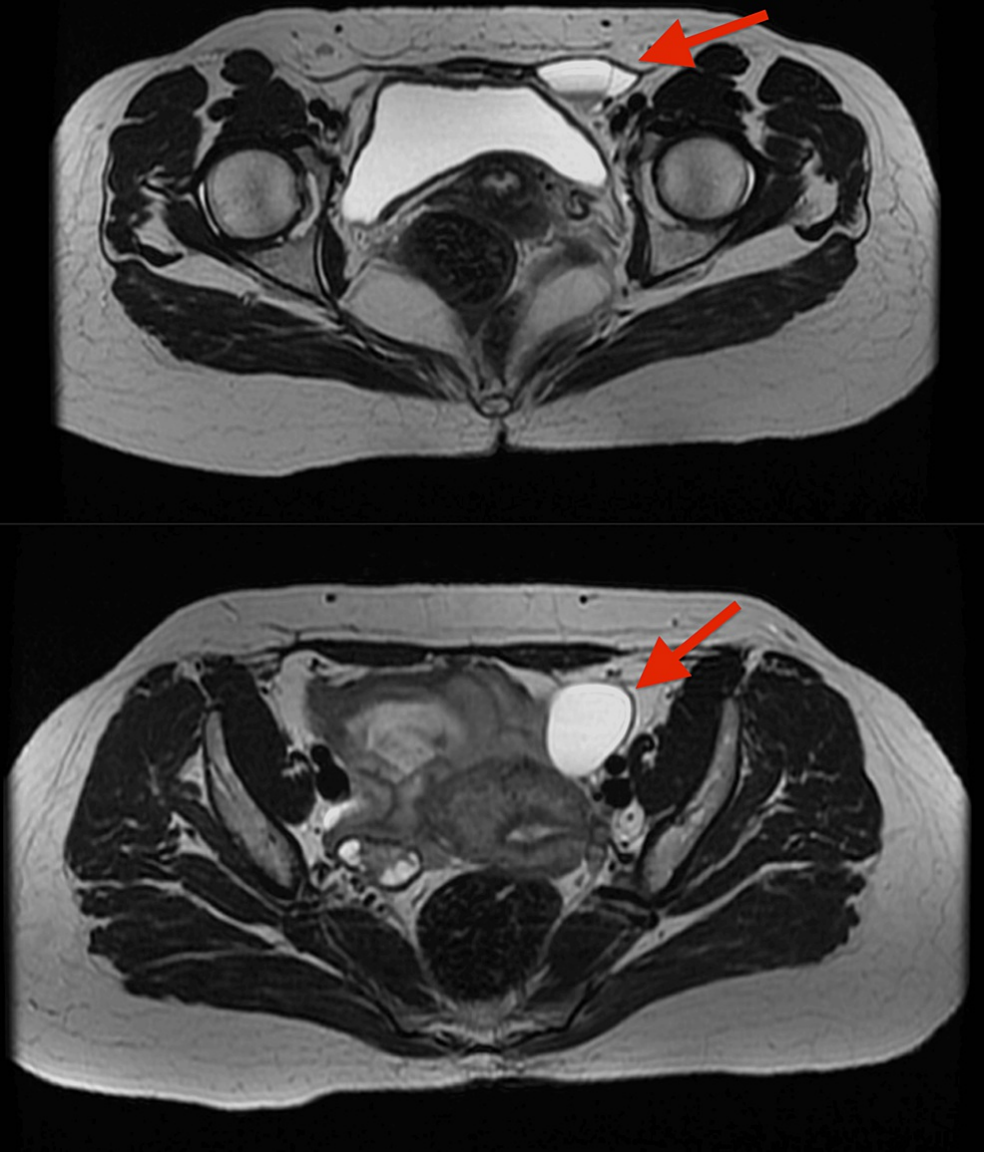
MRI imaging of the pelvis demonstrating a well-defined T2 hyperintense cystic lesion in the pelvis with extension into the inguinal canal (top) with intraperitoneal extension leading to the uterus (bottom)
